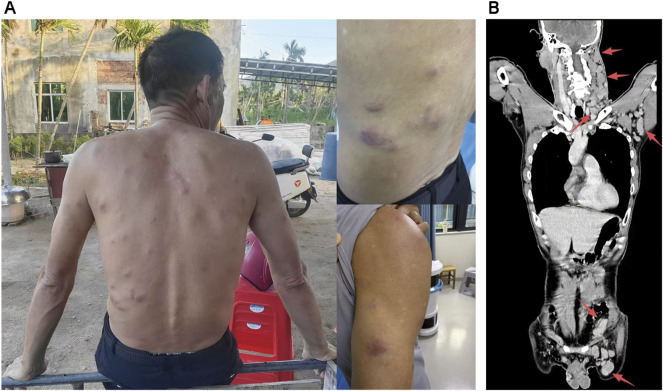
Clinical appearance and imaging findings before treatment. (A) Multiple subcutaneous nodules on the patient’s back, trunk and upper limbs. (B) CT imaging revealing diffuse lymphadenopathy involving cervical, supraclavicular, axillary, retroperitoneal, pelvic, and inguinal regions.
